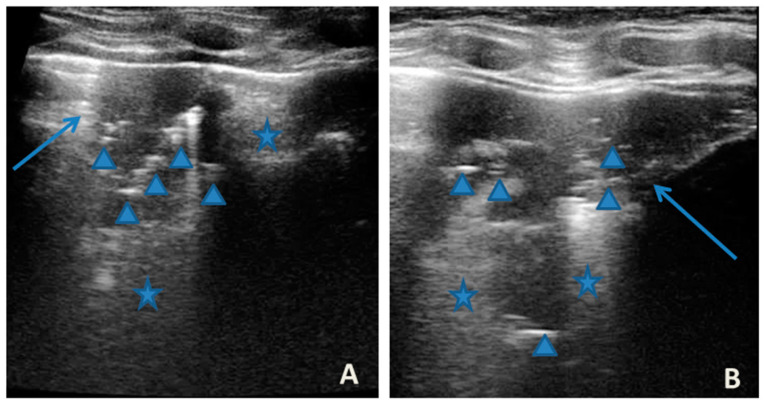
Grayscale lung ultrasound examination shows: (A): on the right anterior field, mainly in the mid-apical area, consolidation of atelectasis origin (arrow) with static air bronchograms (punctate), and parallel to each other (arrowheads), associated with sonographic interstitial syndrome (SIS), represented by coalescent vertical artefacts or B-lines (asterisks); (B): on the left paracardiac site, consolidation of about 2.5–3 cm of atelectasis nature (arrow) with static air bronchograms (punctate) (arrowheads), associated with sonographic interstitial syndrome (SIS), represented by coalescent vertical artefacts or B-lines (asterisks).
